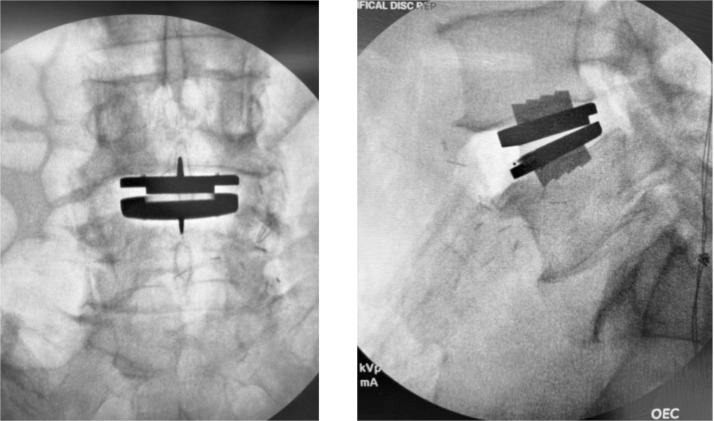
Anteroposterior (AP) and Lateral intraoperative fluoroscopic radiographs following completion of the anterior phase total disc replacement implantation, demonstrating final positioning of the Prodisc L artificial disc replacement implant.
